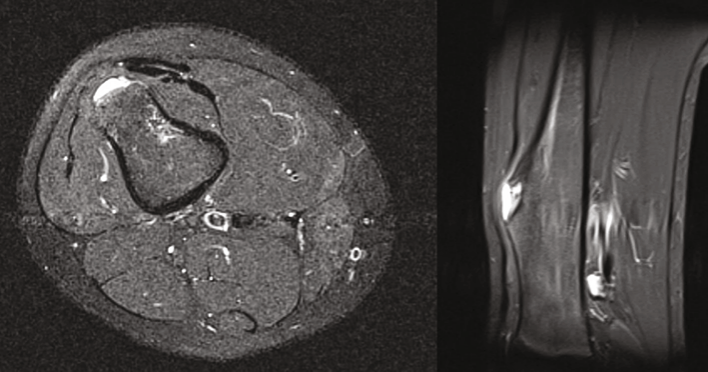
Magnetic resonance images (MRI) (machine settings: thickness 5.0 mm, fat-suppressed axial, and short-T1 inversion recovery (STIR) sagittal) demonstrating a distal femoral lesion consistent with likely osteochondroma.
